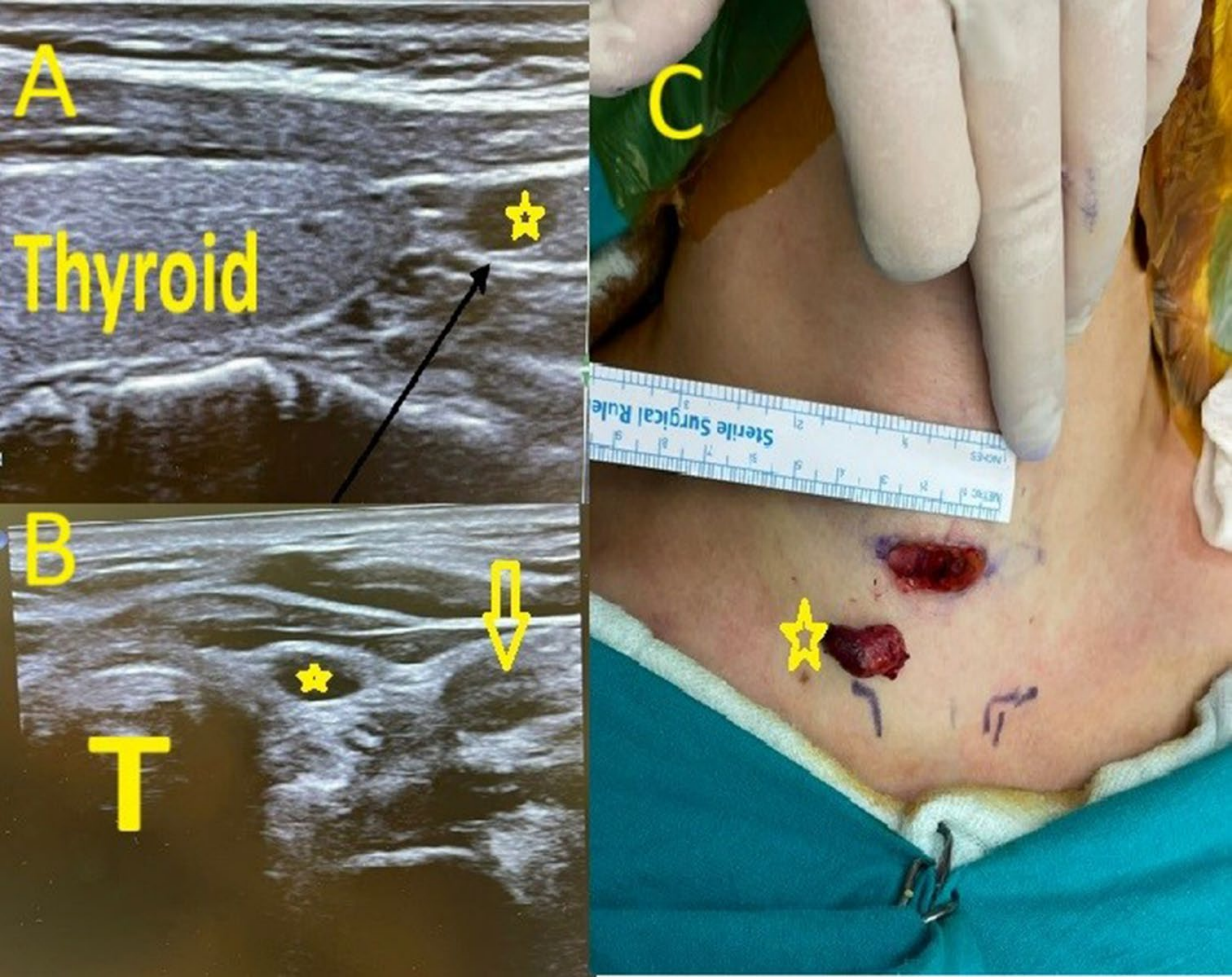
A left inferior parathyroid adenoma. A: Neck ultrasonography( surgeon-performed) sagittal section, * left inferior parathyroid adenoma. B: Neck ultrasonography axial section, * left inferior parathyroid adenoma, T trachea, yellow arrow left common carotid arteria. C: Intraoperative, * parathyroid adenoma
